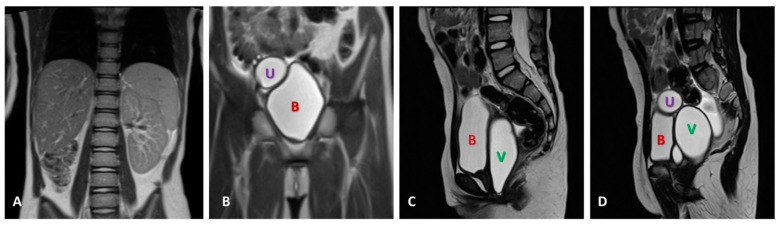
MRI abdominal and pelvic images. (A). Absence of the right kidney, with contralateral hypertrophic kidney (frontal view). (B). Distended urinary bladder (B) and right uterine cavity (U) filled with fluid (frontal view). (C). Distended right hemivagina (V), visible behind the bladder (sagittal view). (D). Hydrometrocolpos (sagittal view).
